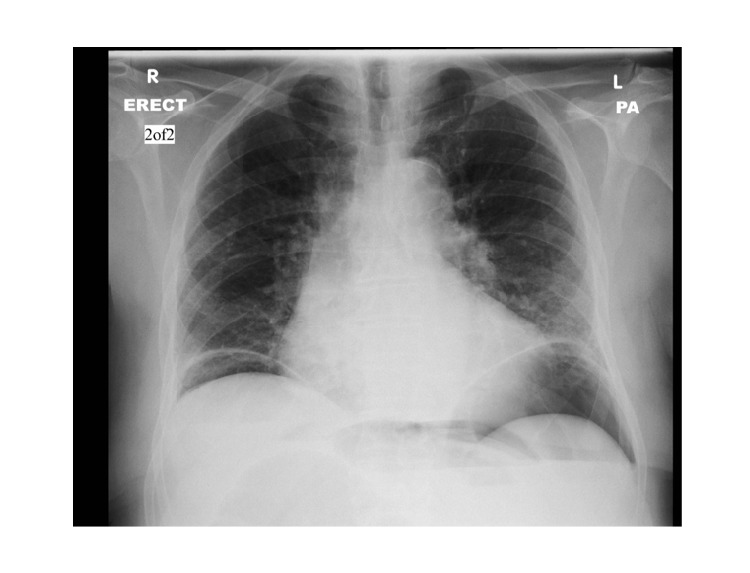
Chest X-ray revealing free intraperitoneal air under the diaphragm.
